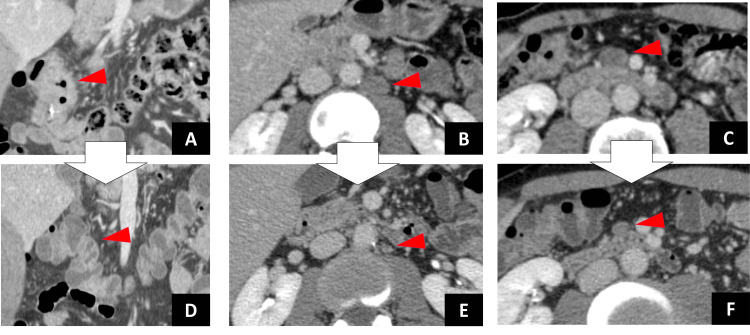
Computed tomography (CT) images. CT images obtained before preoperative chemotherapy: (A) wall thickening at the transverse colon (arrowhead); (B) isolated swollen lymph nodes along the abdominal aorta below the left renal vein (19 mm) (arrowhead); and (C) isolated swollen lymph node (17 mm) (arrowhead). CT images obtained after the administration of six courses of mFOLFOX6 plus bevacizumab: (D) wall thickening not apparent (arrowhead); (E) para-aortic lymph node metastases not apparent (6 mm) (arrowhead); and (F) mesocolon lymph node metastases not apparent (11 mm) (arrowhead).
